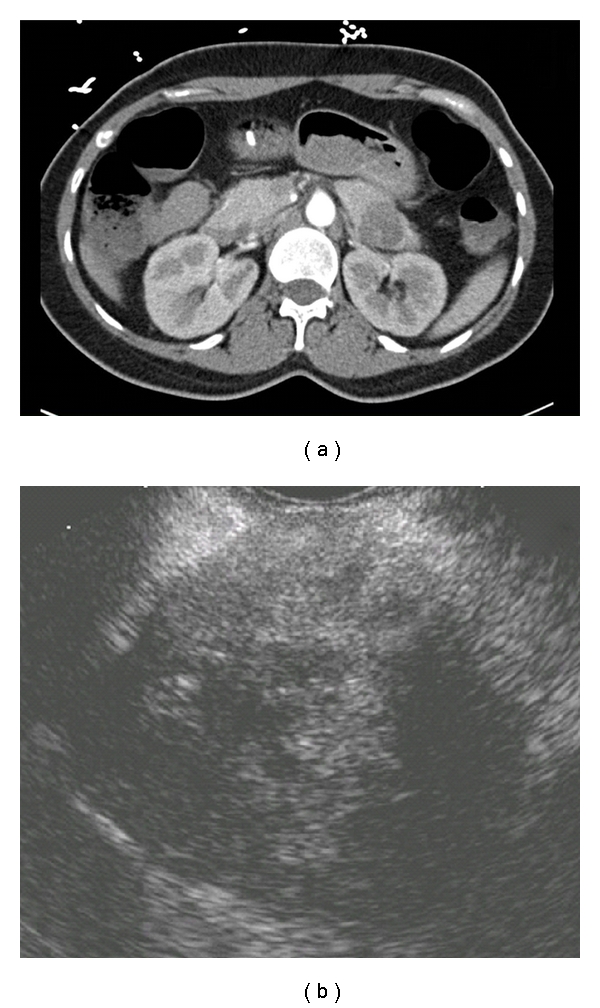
Solid pseudopapillary neoplasm: (a) Typical CT and (b) EUS appearance of solid and cystic components.
